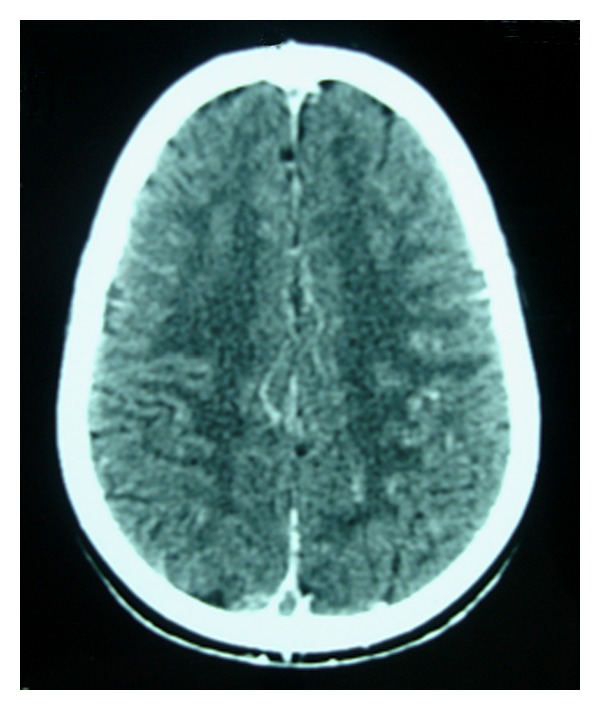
A noncontrast CT scan of brain in axial section showing hyperdense changes (the empty delta sign) consistent with sinovenous thrombosis of *t* posterior part of the sagittal sinus.
